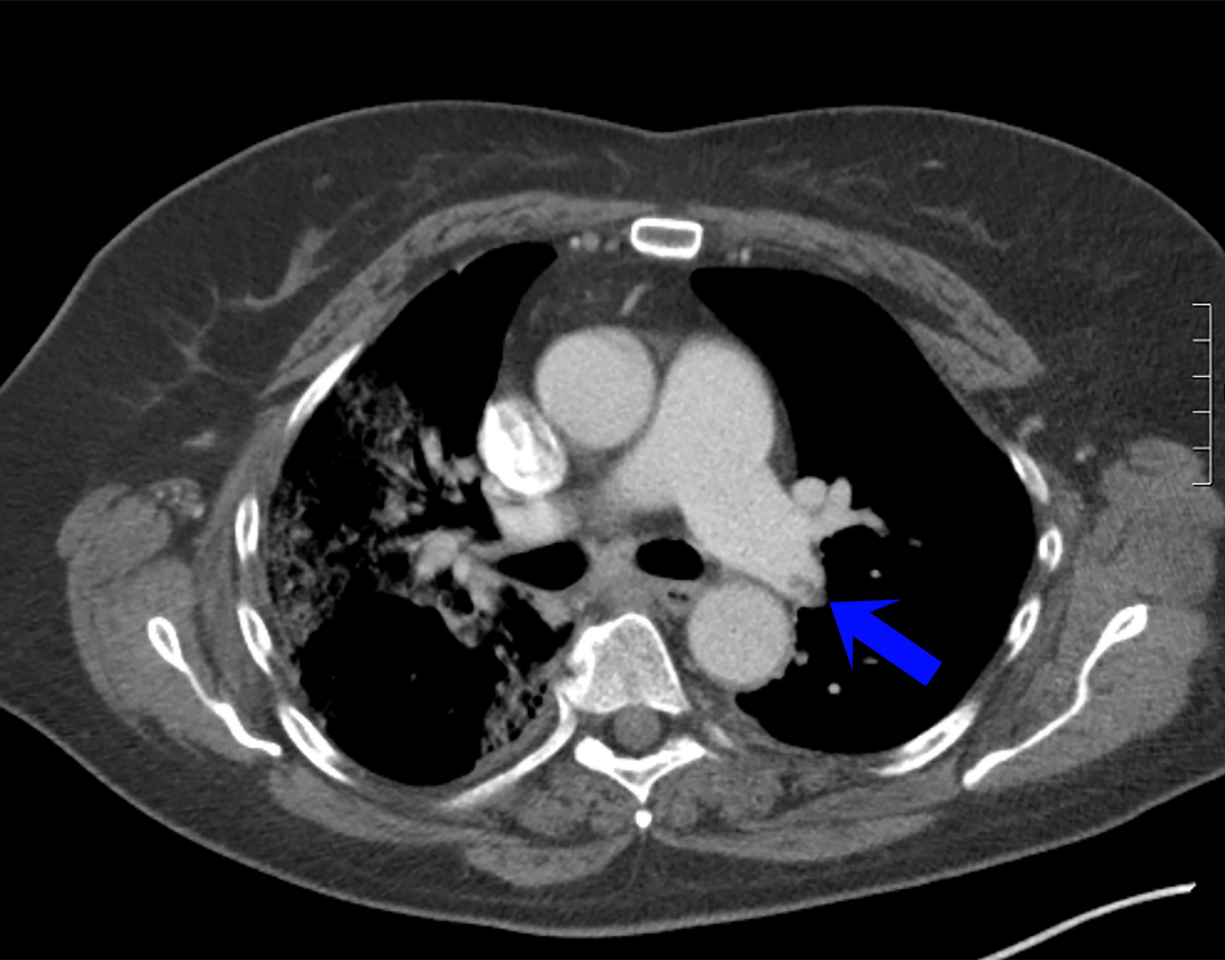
Chest CT showing bilateral ground-glass opacities with crazy paving patterns worse on the right than left, a left upper lobe pulmonary embolism with associated right heart strain, and an enlargement of the main pulmonary artery
